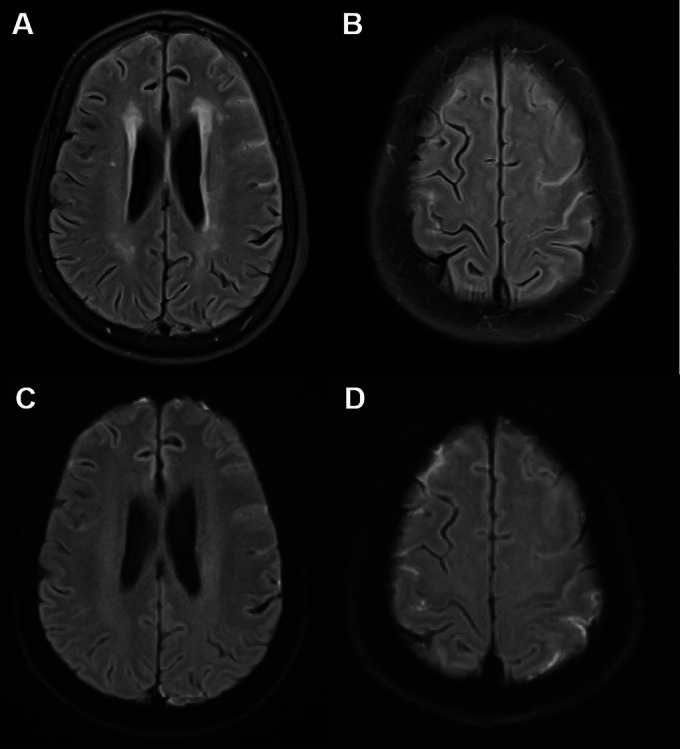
Axial FLAIR MRI sequences (A and B) and DWI (C and D) showing hyperintense lesions in the bilateral frontal–parietal and left temporal leptomeninges.
